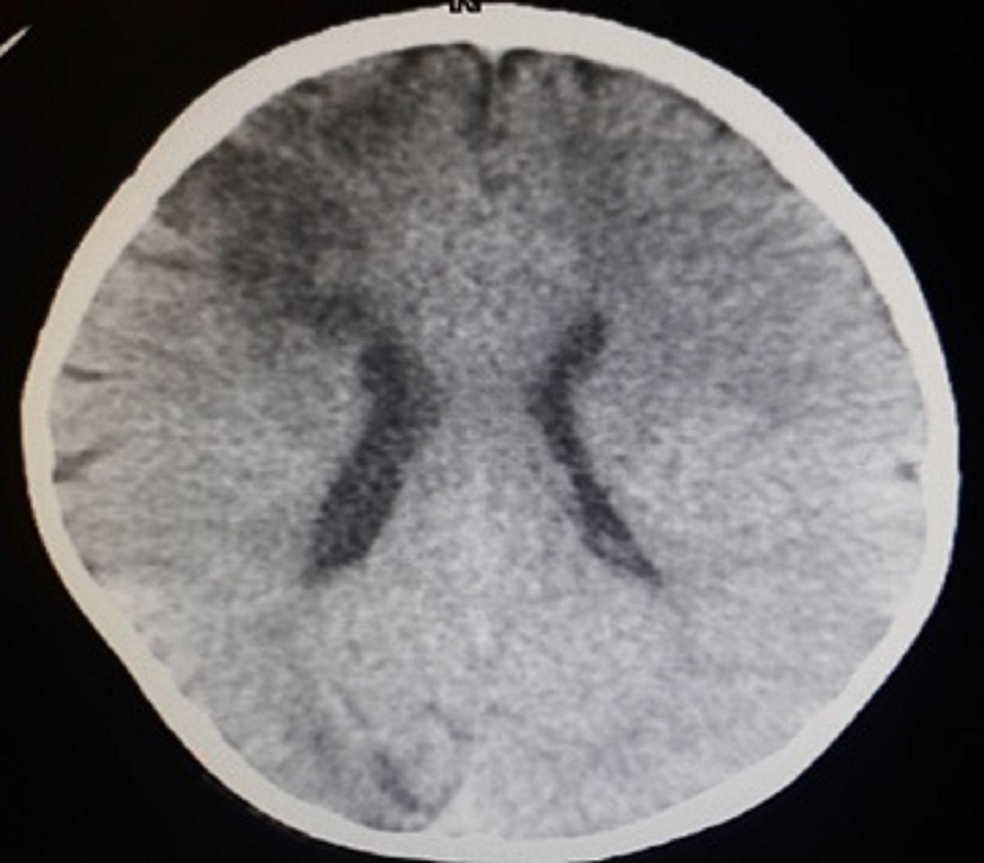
Non-contrast axial brain computed tomography (CT) image showing a hypodense area in the right hemisphere due to an old infarction.
